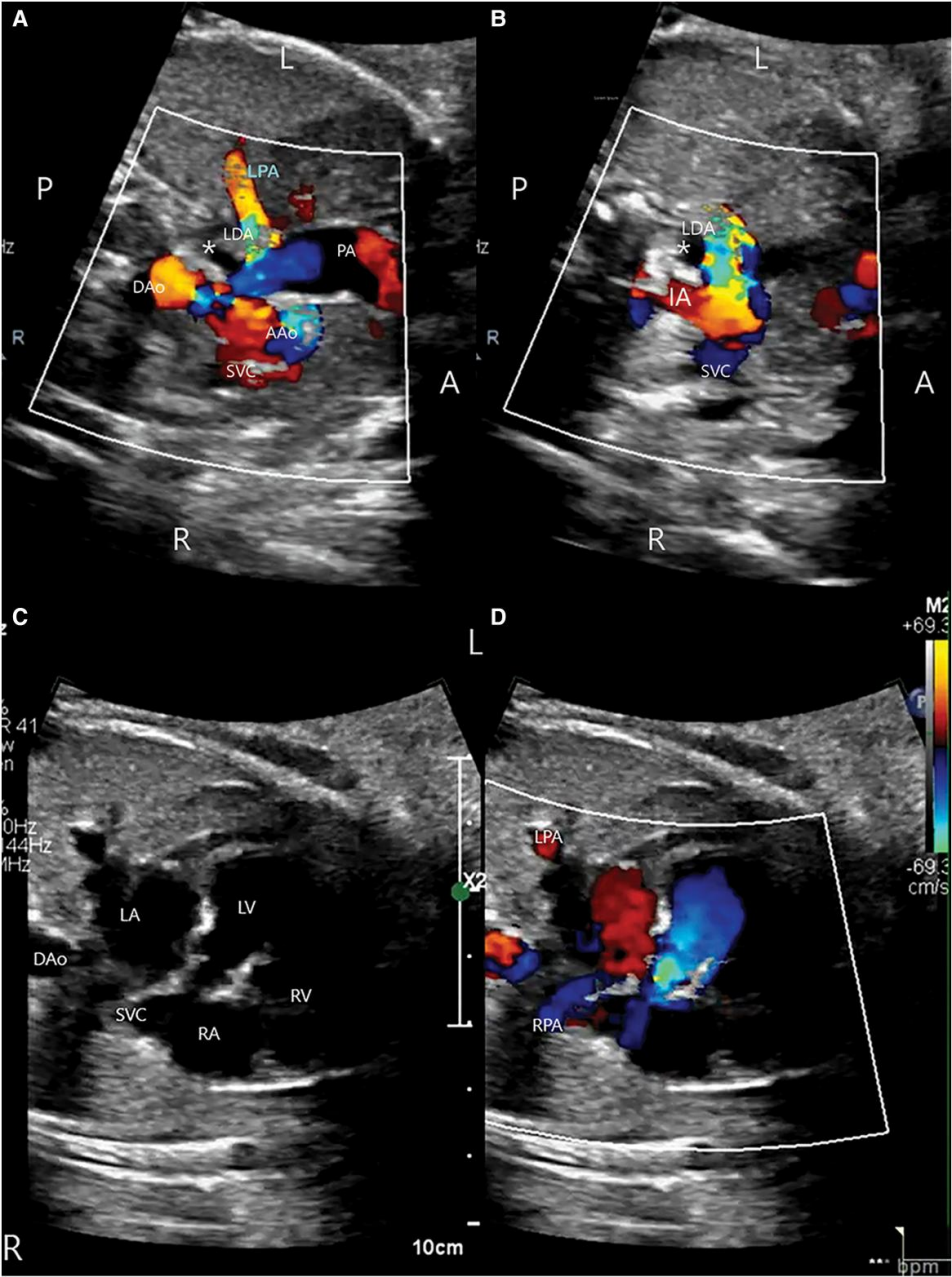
(*A* and *B*) Antenatal echocardiography at 32 weeks gestational age: a sweep depicting blood flow from PA to LDA to the isolated LPA. The LDA arises from the base of the left IA. (*C* and *D*) Sweep from four-chamber view, to three-vessel view, to the neck vessels, demonstrates that the LPA and RPA are discontinuous (see Supplementary material online, *Video S1A* and *B*).
